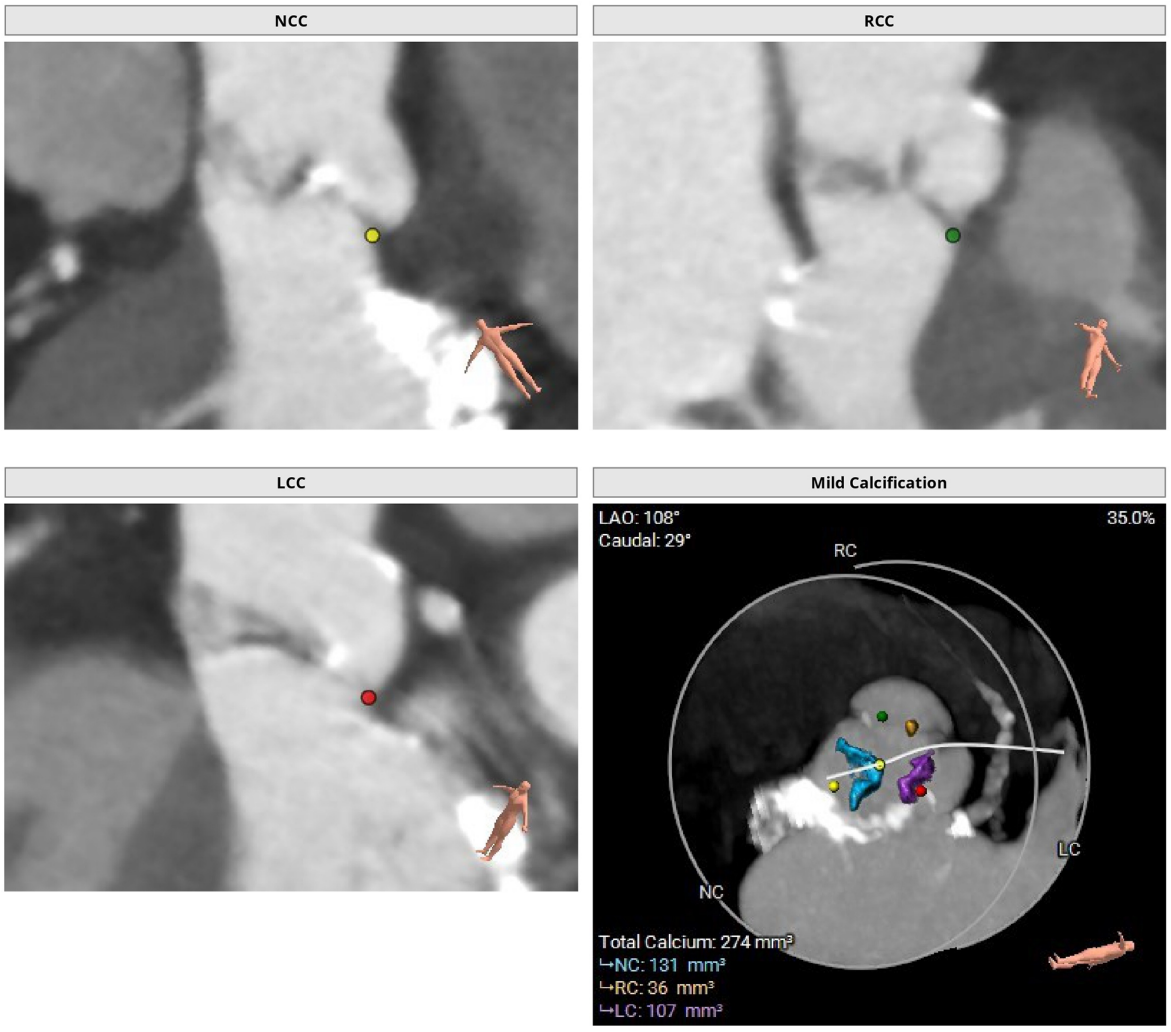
Preoperative thoraco-abdominal CT images were analyzed using 3mensio Structural Heart software (Pie Medical Imaging, the Netherlands). Preoperative thoraco-abdominal CT images showed a tricuspid aortic valve with mild valvular calcifications.
